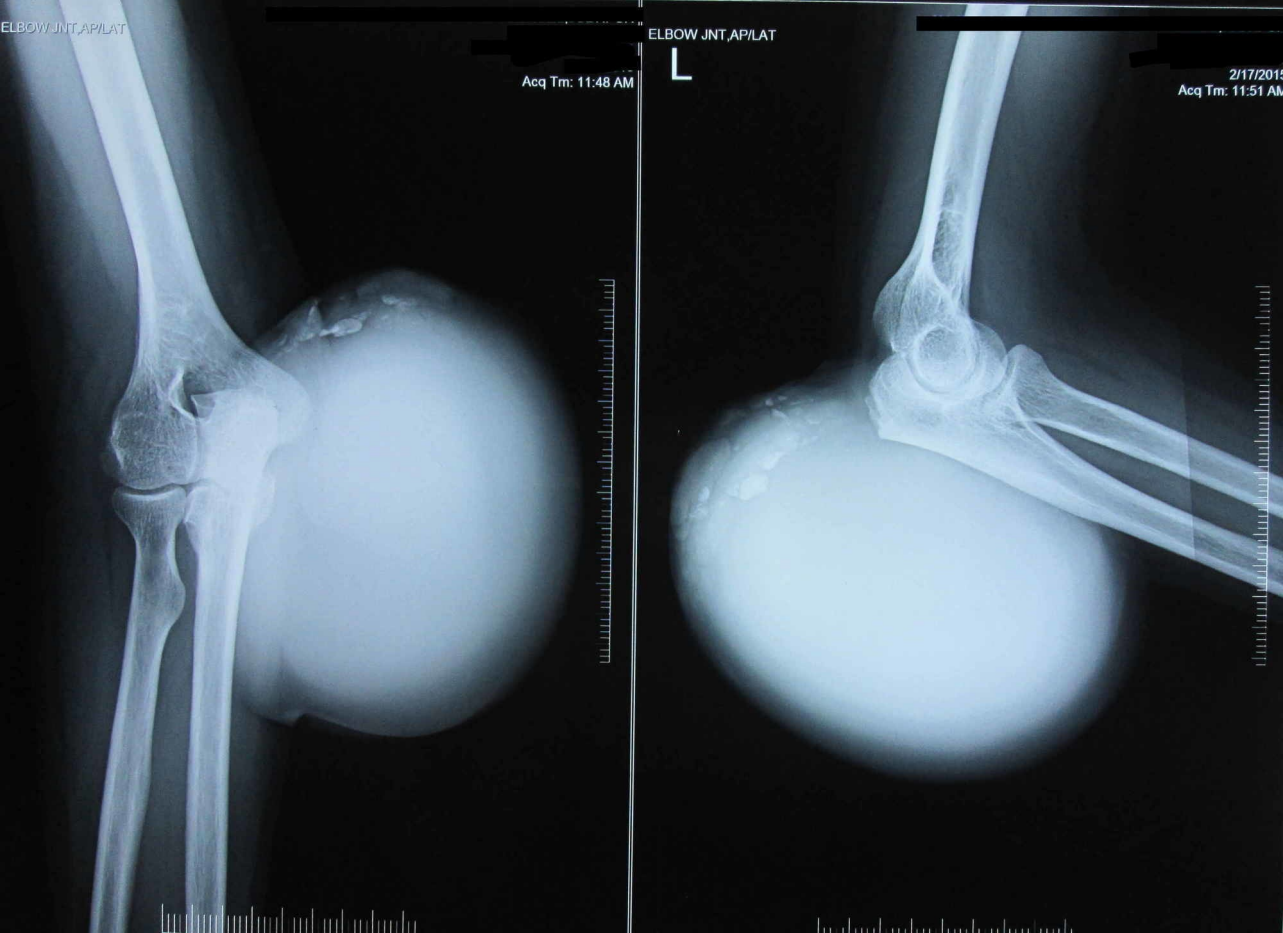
AP and lateral radiograph of the left elbow showing a huge soft tissue shadow with calcifications.
